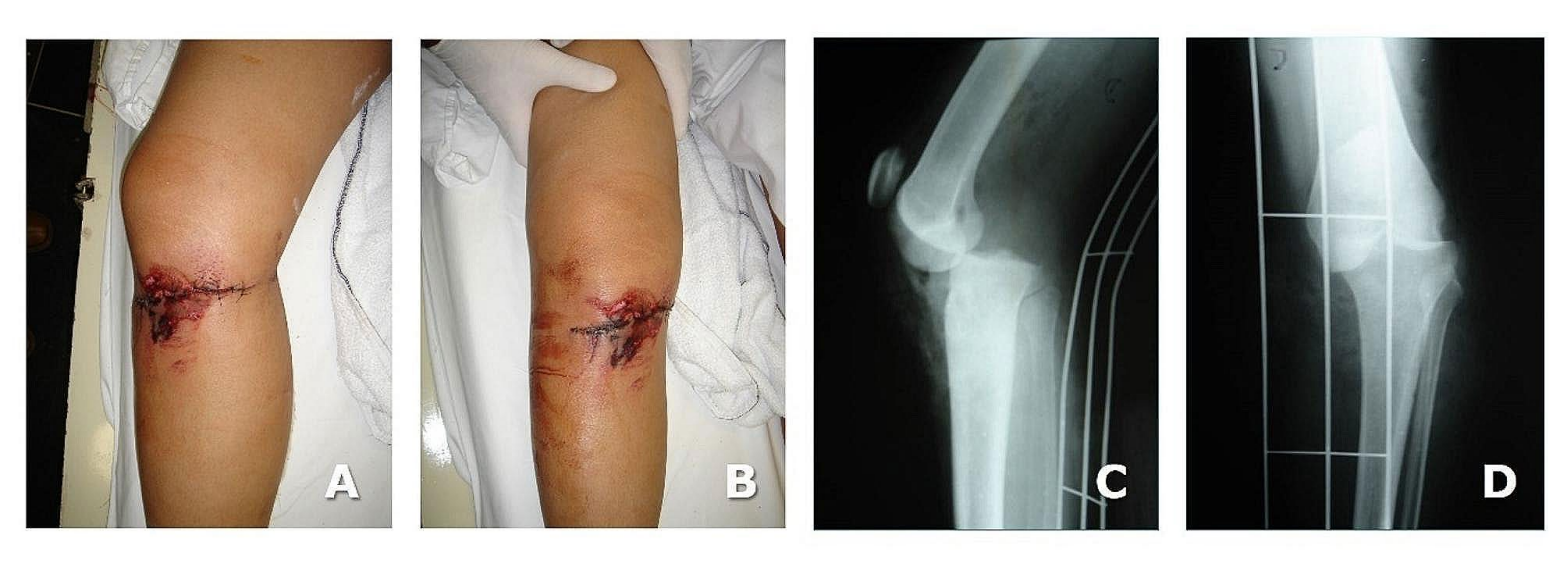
Clinical appearance of the right knee, sutured skin laceration on the day of injury (A and B), and (C) lateral and (D) anteroposterior radiographs
